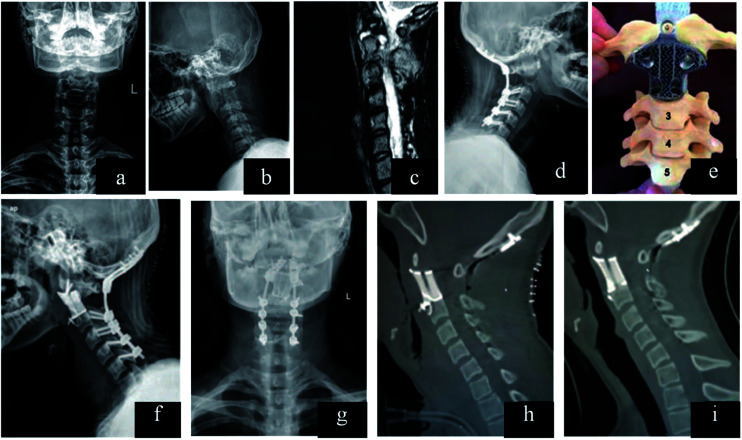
(a–c) Lesion and its boundary located in the preoperative X-ray and MRI. (d) After resection of the posterior C2 elements and fixation in the lateral X-ray. (e) Model showed that how the 3D vertebral body was inserted between C1 and C3 in the second surgery. (f and g) Anteroposterior and lateral X-ray after the second surgery. (h) Sagittal reconstruction after surgery and (i) at the 1 year follow-up demonstrating implant osseointegration without implant failure and local recurrence of the tumor. This figure has been reproduced from ref. 162 with permission from Wolters Kluwer.
